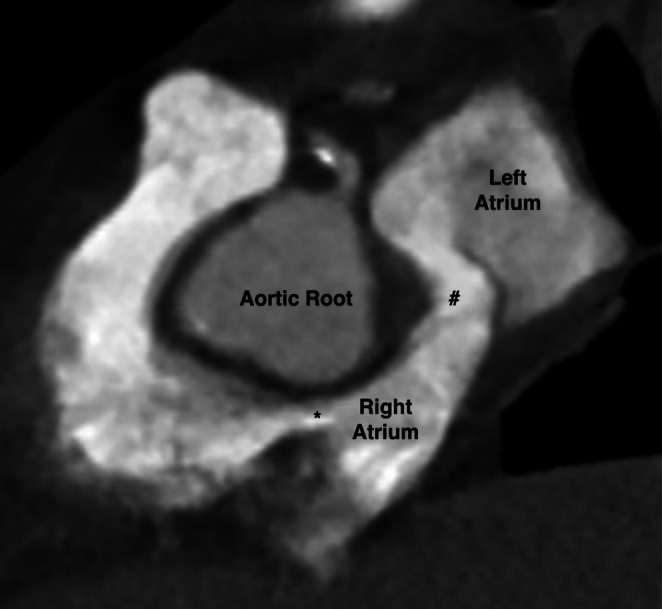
CT image demonstrating the septum primum of the interatrial septum (#) bowing into the left atrium with contrast flowing through the PFO. Note the compression of both the right and left atrium and the tricuspid annulus (*).
